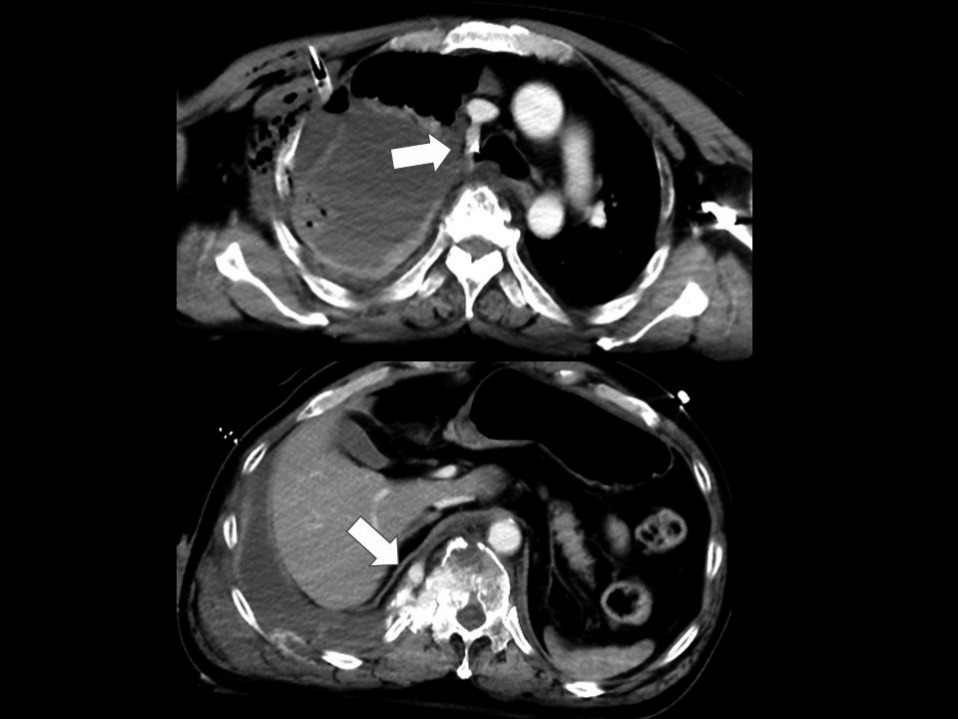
Enhanced chest computed tomography after tentative thoracotomy Bleeding from the azygos arch was controlled (upper arrow) but hemorrhaging from the inferior azygos vein near the thoracic vertebral fracture remained (lower arrow).
